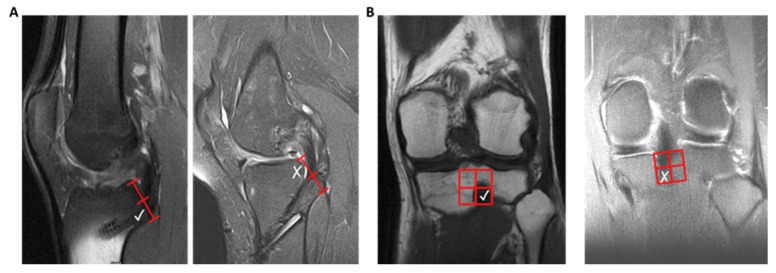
MRI images demonstrating the evaluation method of ideal tibial tunnel opening. (A) red line divided retro-spinal surface to superior and inferior half; left: ideal tunnel located in the inferior half; right: non ideal tunnel located in superior half; (B) Red frame demonstrated the coronal plane of tibial footprint, and its midline divided the lateral and medial half, left: ideal tunnel located in lateral half; right: non ideal tunnel located in medial half. The white check mark indicates ideal position, whereas the white cross symbol indicates non ideal position.
